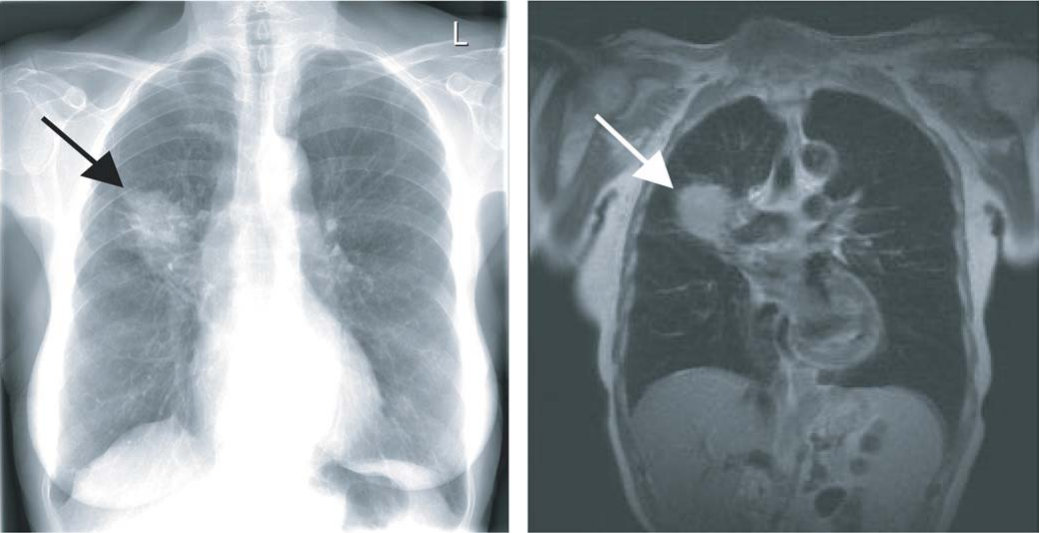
Baseline chest x-ray (left) and MRI scan (right) of patient 1. Note the large tumor originating from the upper right lobe of the lung (arrows). The patient was resected with an upper bilobectomy (pT2 pN2, IIIA) and systematic lymphadenectomy and the diagnosis of NSCLC (adenocarcinoma) was confirmed.
